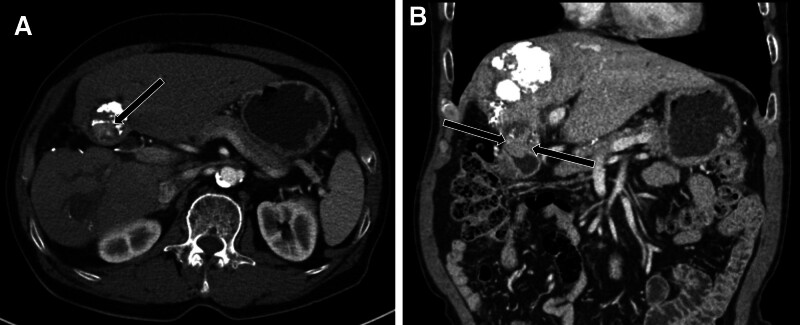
Preprocedural CT images obtained before the 5th TACE. (A) Axial plane arterial phase images of a viable tumor in S4b of the liver (arrow). (B) Coronal reformatted images of a diminished fat plane between the tumor and the duodenal 1st portion (arrows) with suspected tumor invasion. CT = computed tomography, TACE = transarterial chemoembolization.
